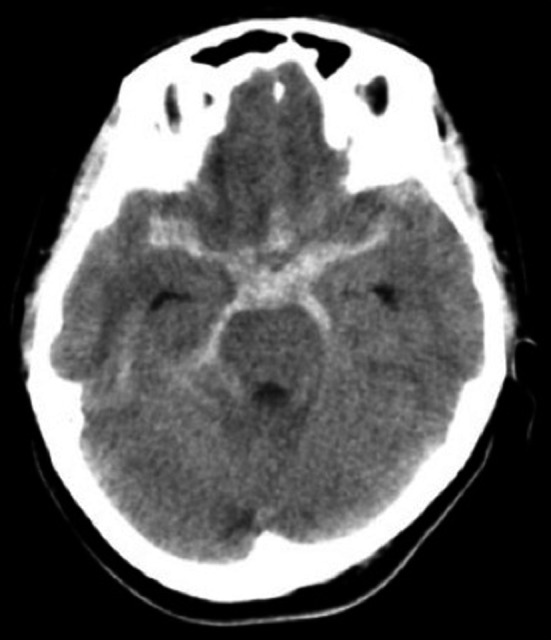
Axial computed tomography showing a diffuse subarachnoid hemorrhage in the basal cistern that is relatively prominent on the right side.
